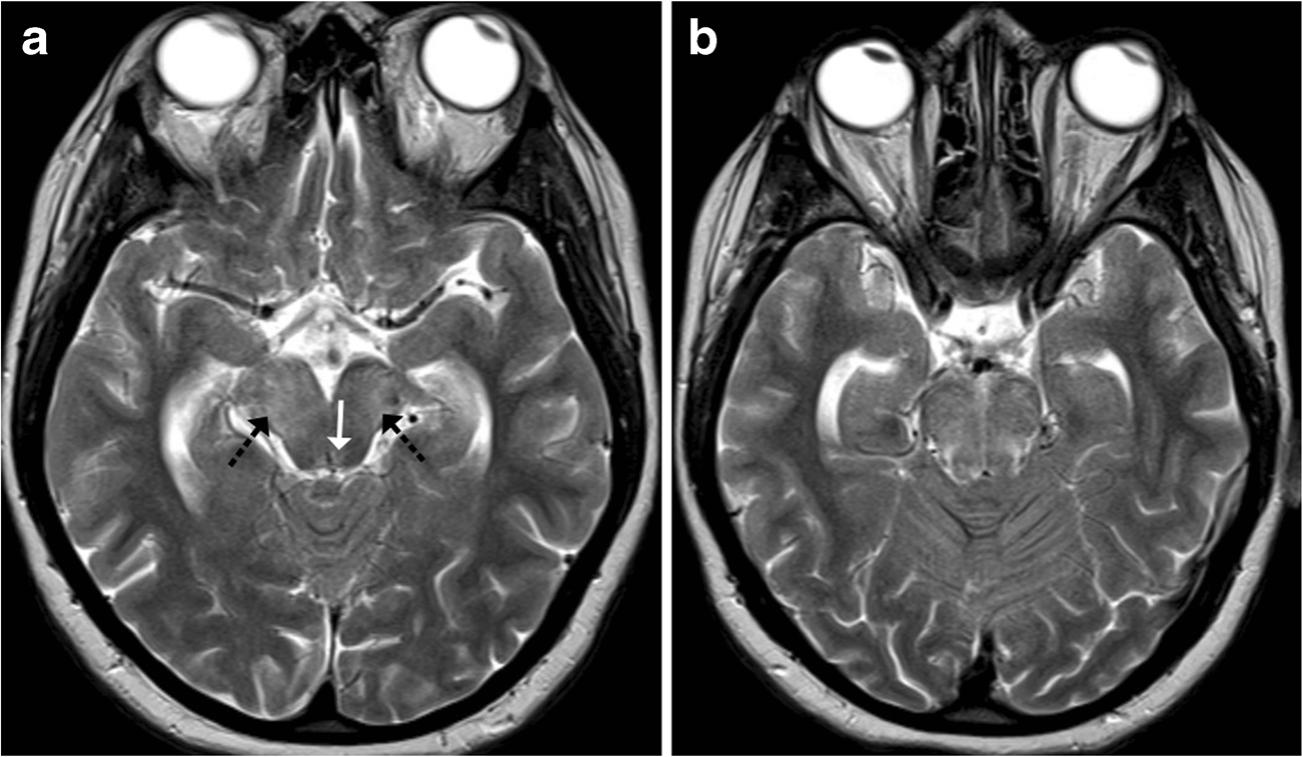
A 16-year-old female with neuromyelitis optica: a–b Axial T2-weighted images show hyperintense signal involving the tegmentum (*dotted arrow*), periaqueductal region (*arrow*) of the midbrain and pons in a diffuse pattern
